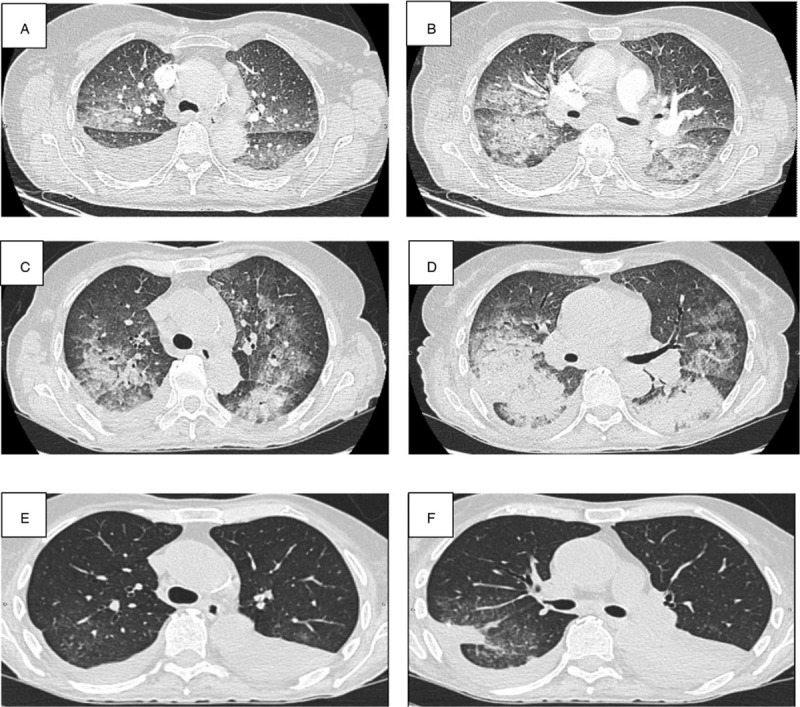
High resolution CT. Chest CT-scan at the initial presentation (A, B), after exacerbation (C, D), and after rituximab treatment (E, F). CT = computed tomography
